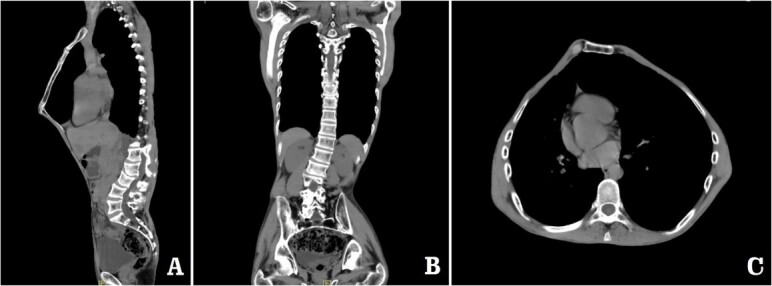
Computed tomography examination before operation. (A) Sagittal view; (B) coronal view; and (C) sectional view.
